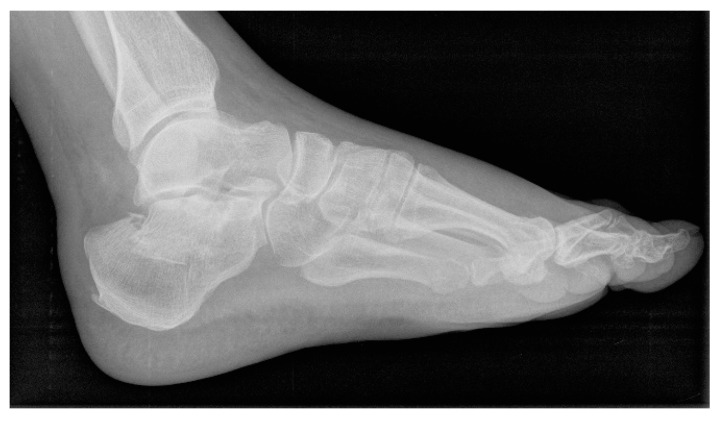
Lateral view radiograph of a calcaneal fracture.
